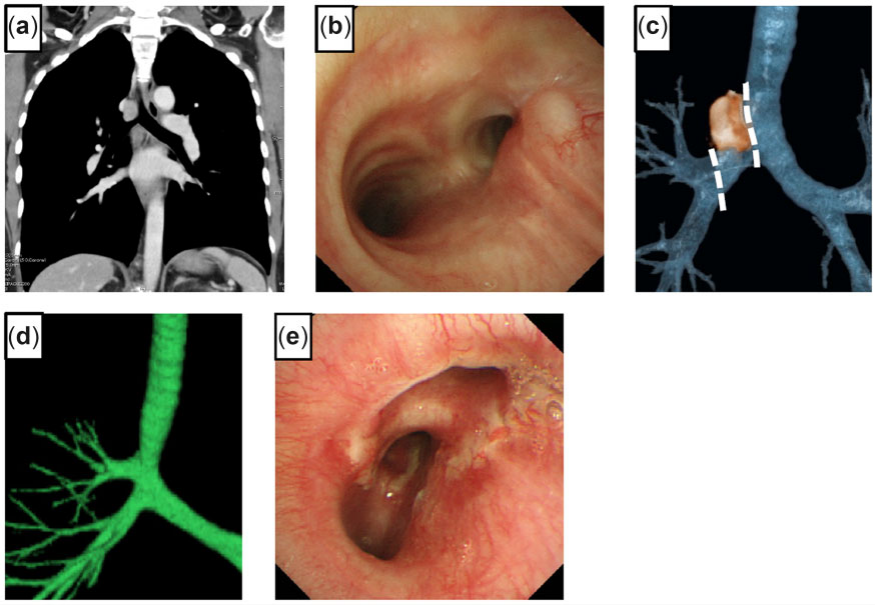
Chest computed tomography in a coronal view shows a tumour around the proximal right main bronchus (a). Bronchoscopy shows the membranous part of the right lateral wall of the lower trachea pressed by a tumour (b). The bronchus occupied by a tumour was divided obliquely at the proximal and distal sides (c). A 3-dimensional computed tomography scan shows airway lumen patency of the anastomosis (d). Bronchoscopy 2 months after the operation shows healing of the anastomosis (truncus intermedius).
